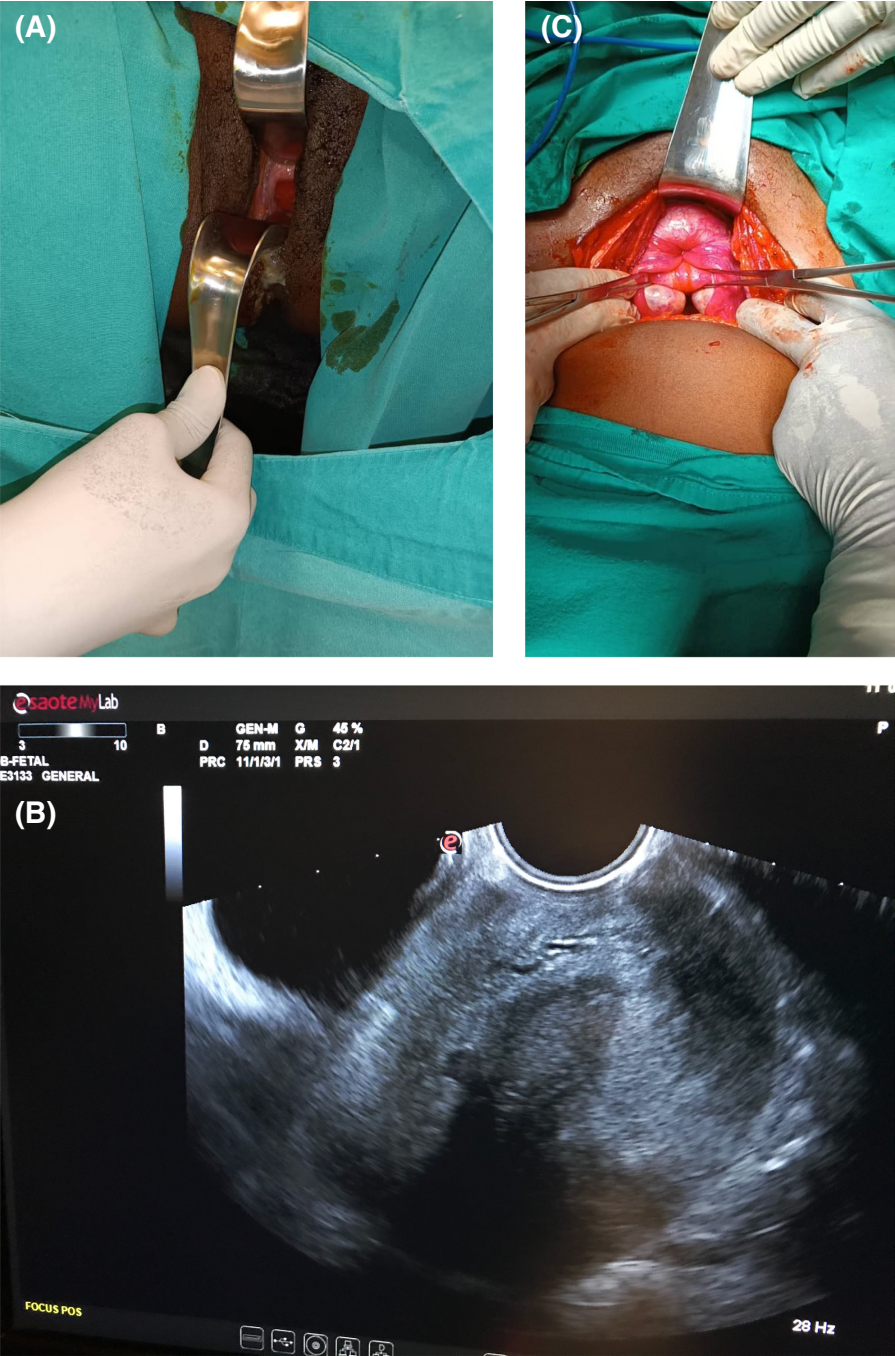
(A) Image of bleeding uterus on preoperative vaginal examination. (B) The uterus is seen to be inverted in the transvaginal ultrasonographic image. (C) Kissing ovaries and tubes in intraoperative pelvic examination (uterus cannot be clearly observed) (flowerwave apperance).
